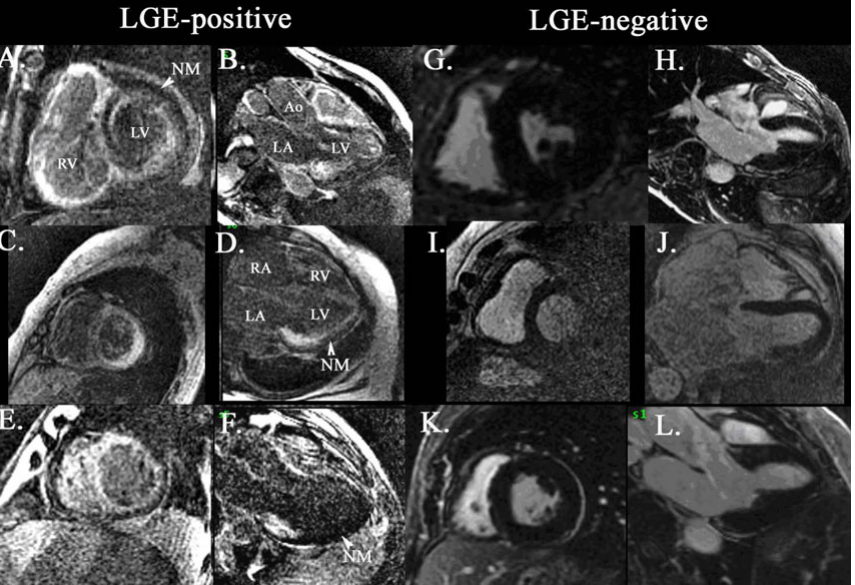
Cardiac MRI. Inversion-recovery prepared segmented gradient echo sequence short axis and long axis images of 3 patients with late gadolinium enhancement (A-B, C-D and E-F) and 3 patients without late gadolinium enhancement (G-H, I-J and K-L). Note the diffuse left ventricular subendocardial enhancement in LGE-positive patients and significant right ventricular involvement in the first two subjects. The dark nulled normal myocardium is located in the subepicardial portion. The dark blood pool signal in LGE-positive subjects is consistent with initial observations by Maceira, et al. [6] that they related to high myocardial uptake and fast blood washout. LV-left ventricle, RV-right ventricle, LA-left atrium, RA-right atrium, Ao-aorta, NM-nulled myocardium.
